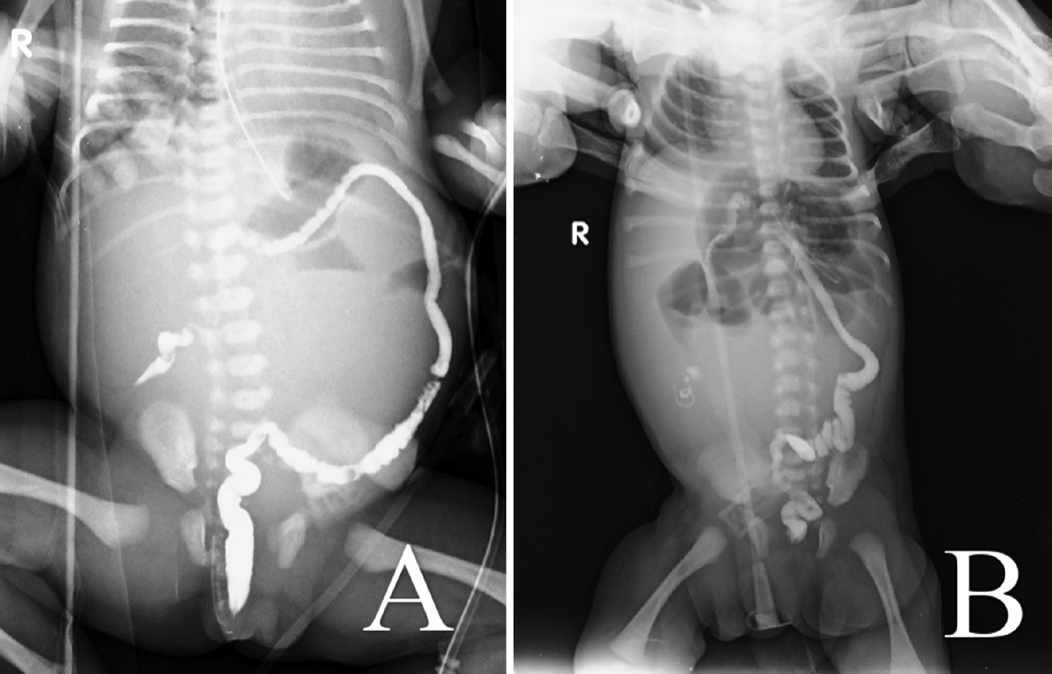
A. Pre-operative and B. Post-operative abdominal radiography of case 2. Pre-operative screening shows the blockage of ileus due to meconium ileus, whereas post-operative screening shows that there is no blockage after surgery.
